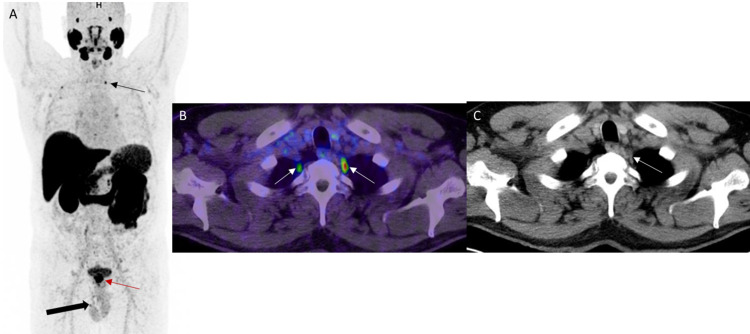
Normal uptake in the stellate ganglia A: MIP image shows uptake in the left stellate ganglion (black thin arrow). Note the pathological uptake in the prostate bed (red arrow). There is mild physiological activity at the testes (black thick arrow). B: PET/CT axial image of the neck and upper chest shows uptake in the bilateral stellate ganglia, left more than right (white arrows). C: CT component of PET/CT shows correlate ovoid-shaped left stellate ganglia (white arrow).
